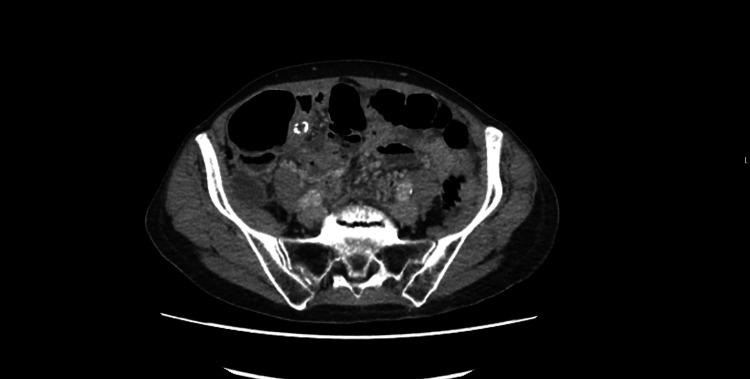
Axial contrast-enhanced CT of the abdomen and pelvis demonstrating a fluid-filled, dilated tubular structure in the right lower abdomen with mural calcification at its base, continuous with the cecum, consistent with a possible appendiceal mucocele.
